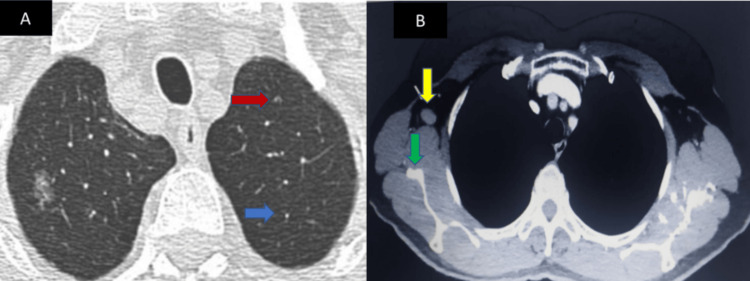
Axial CT scan showing four significant findings highlighted with color-coded arrows Red arrow: significant enlargement of the necrotic mass in the right axillary region; blue arrow: two micronodules in the middle lobe; green arrow: a left basal pulmonary nodule; yellow arrow: mediastinal millimetric lymph node
